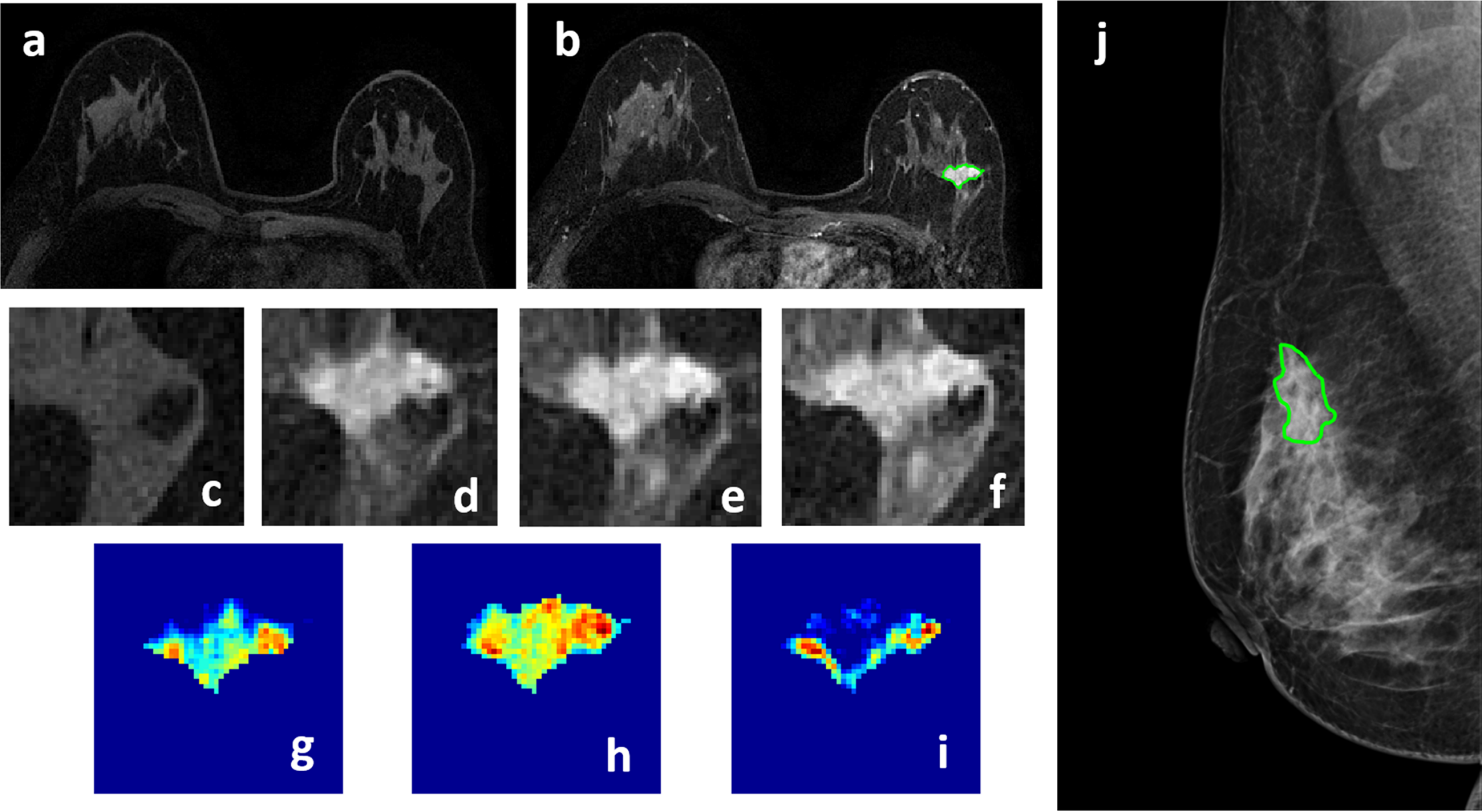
A 50-year-old patient with invasive ductal cancer, showing a strongly enhanced 1.8 x 1.0 cm lesion, with MRI BI-RADS score of 5. (A) F1 Pre-contrast image. (B) F2 post-contrast image. (C–I) Magnified images to demonstrate the margin and internal enhancements within the lesion. (C) F1 pre-contrast, (D) F2 post-contrast, (E) F3 post-contrast, (F) The last F6 post-contrast image. (G) The wash-in signal enhancement map F2-F1, (H) The F3-F1 signal enhancement map, (I) The wash-out F6-F3 map. (J) A mass lesion with spiculation is clearly noted on mammography as BI-RADS 4C, and manually outlined by a radiologist. The radiomics malignancy probability predicted by MRI, mammography, and combined models were: 0.83, 0.77, 0.88, respectively, true positive.
